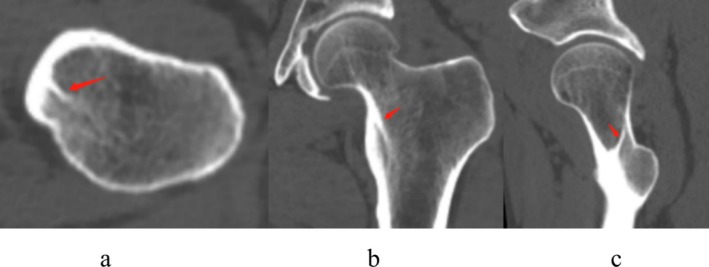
Multiplanar CT visualization of calcar femorale anatomy. (a) Axial view demonstrates the dense cortical projection (red arrow) extending from the posteromedial femoral cortex. (b) Coronal reconstruction shows the orientation of the calcar femorale (red arrow) along the femoral neck axis. (c) Sagittal view demonstrates the calcar femorale's anatomical continuity (red arrow), with its superior aspect merging with the mid‐femoral neck's posteromedial cortex and its inferior aspect blending with the lesser trochanter's cortical bone.
